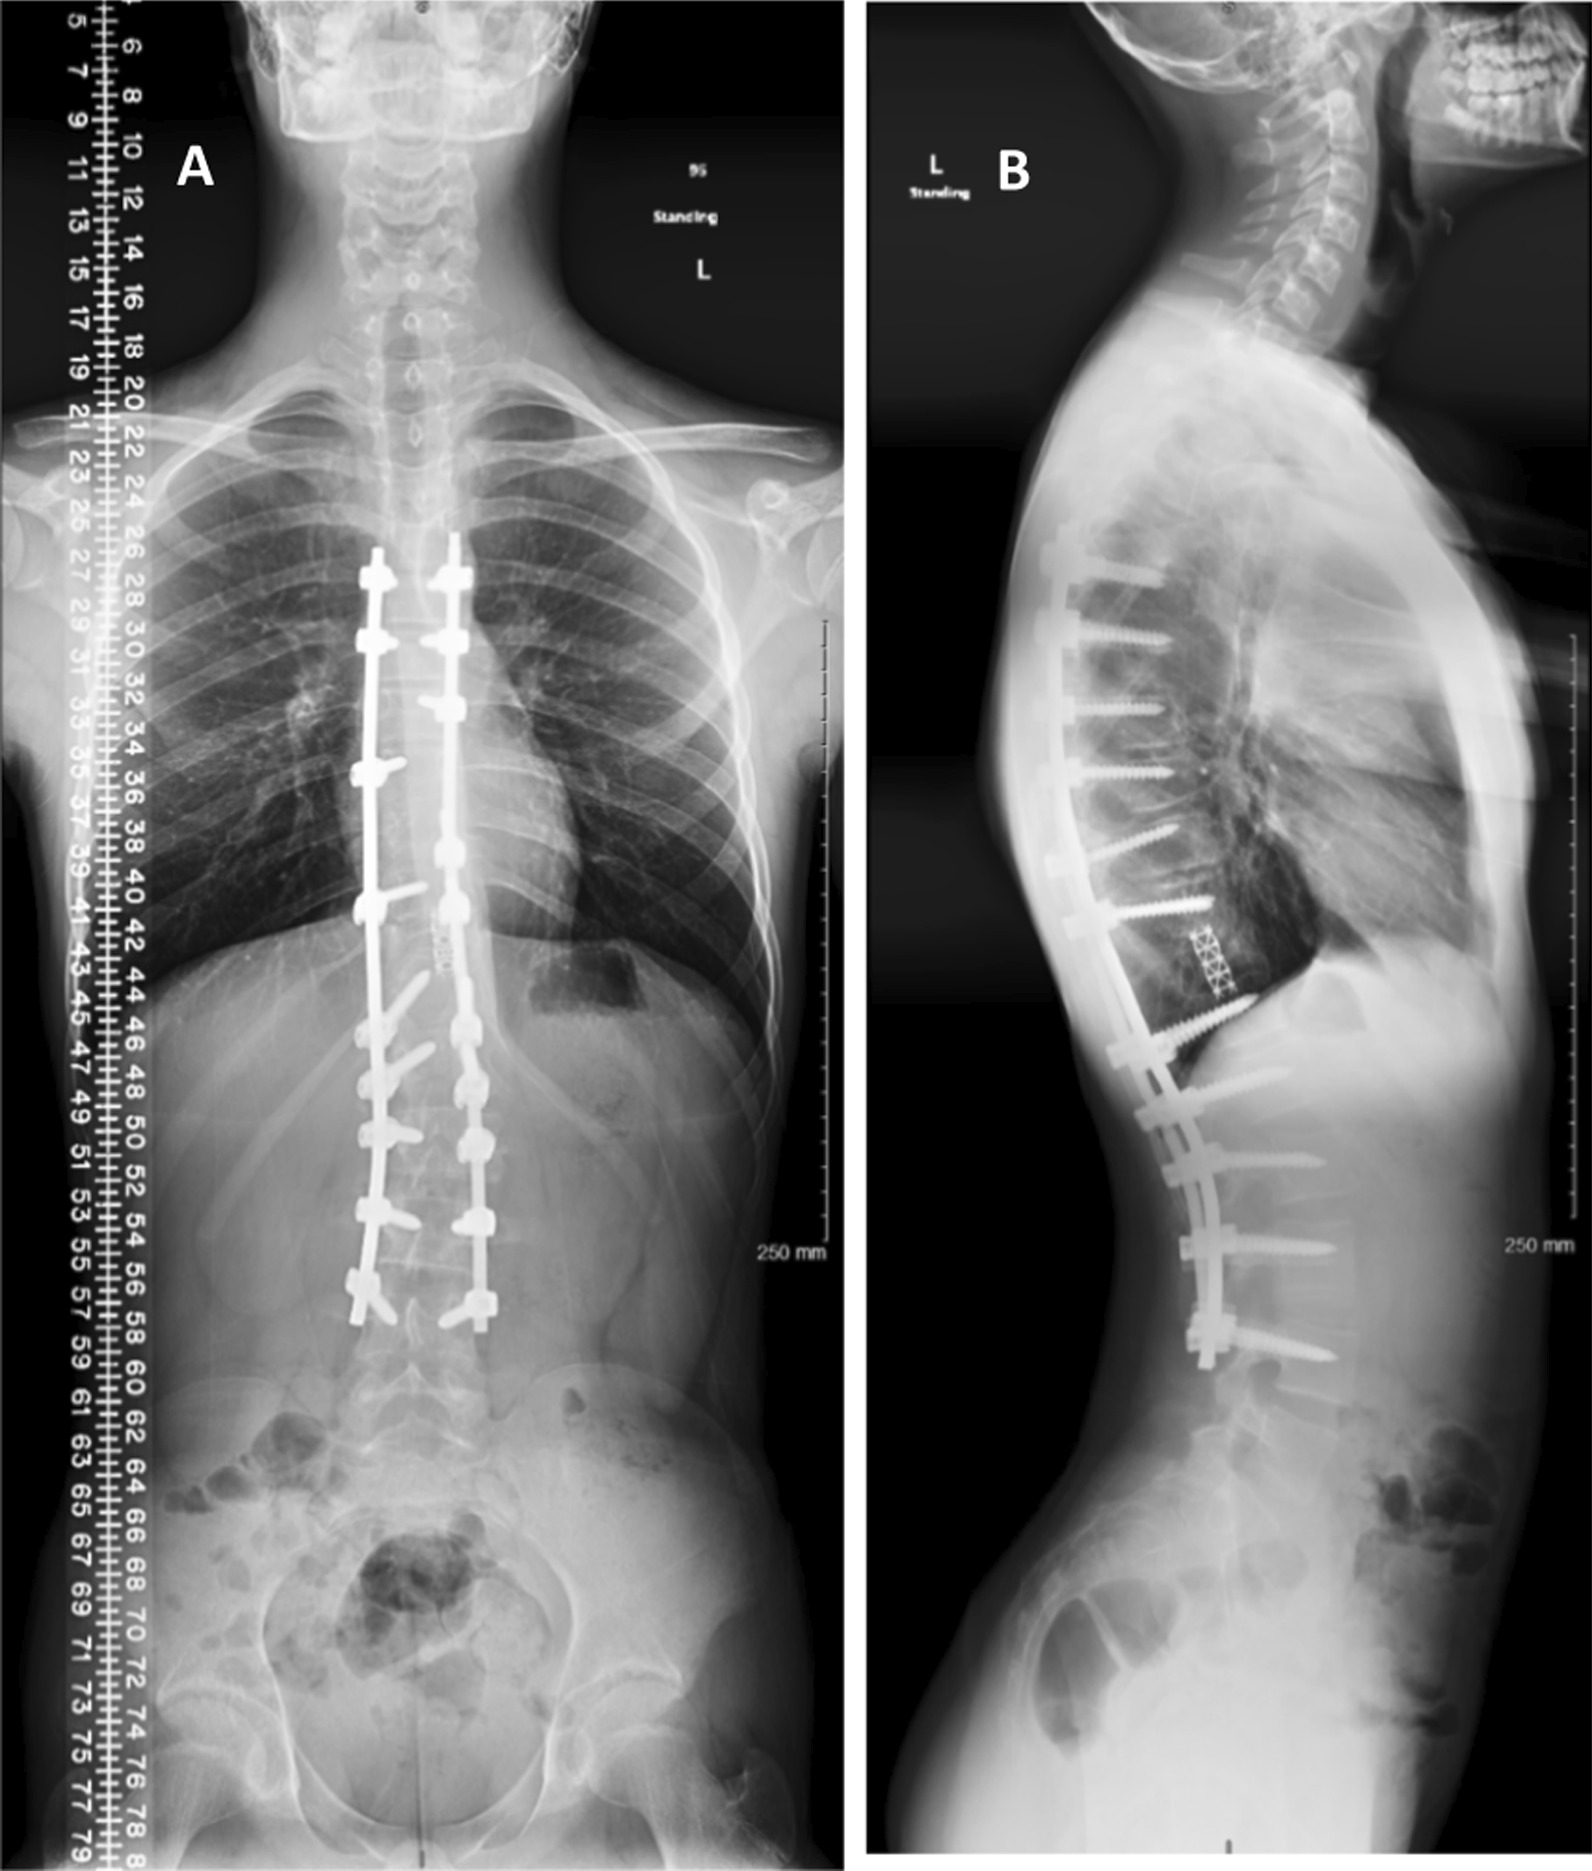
A, B Scoliosis X-ray A AP and B lateral, 1 year post revision surgery showing T11 resected vertebral body with cage placement, and posterior spinal fixation from T5 to L4 with scoliosis improvement and no complication is seen
